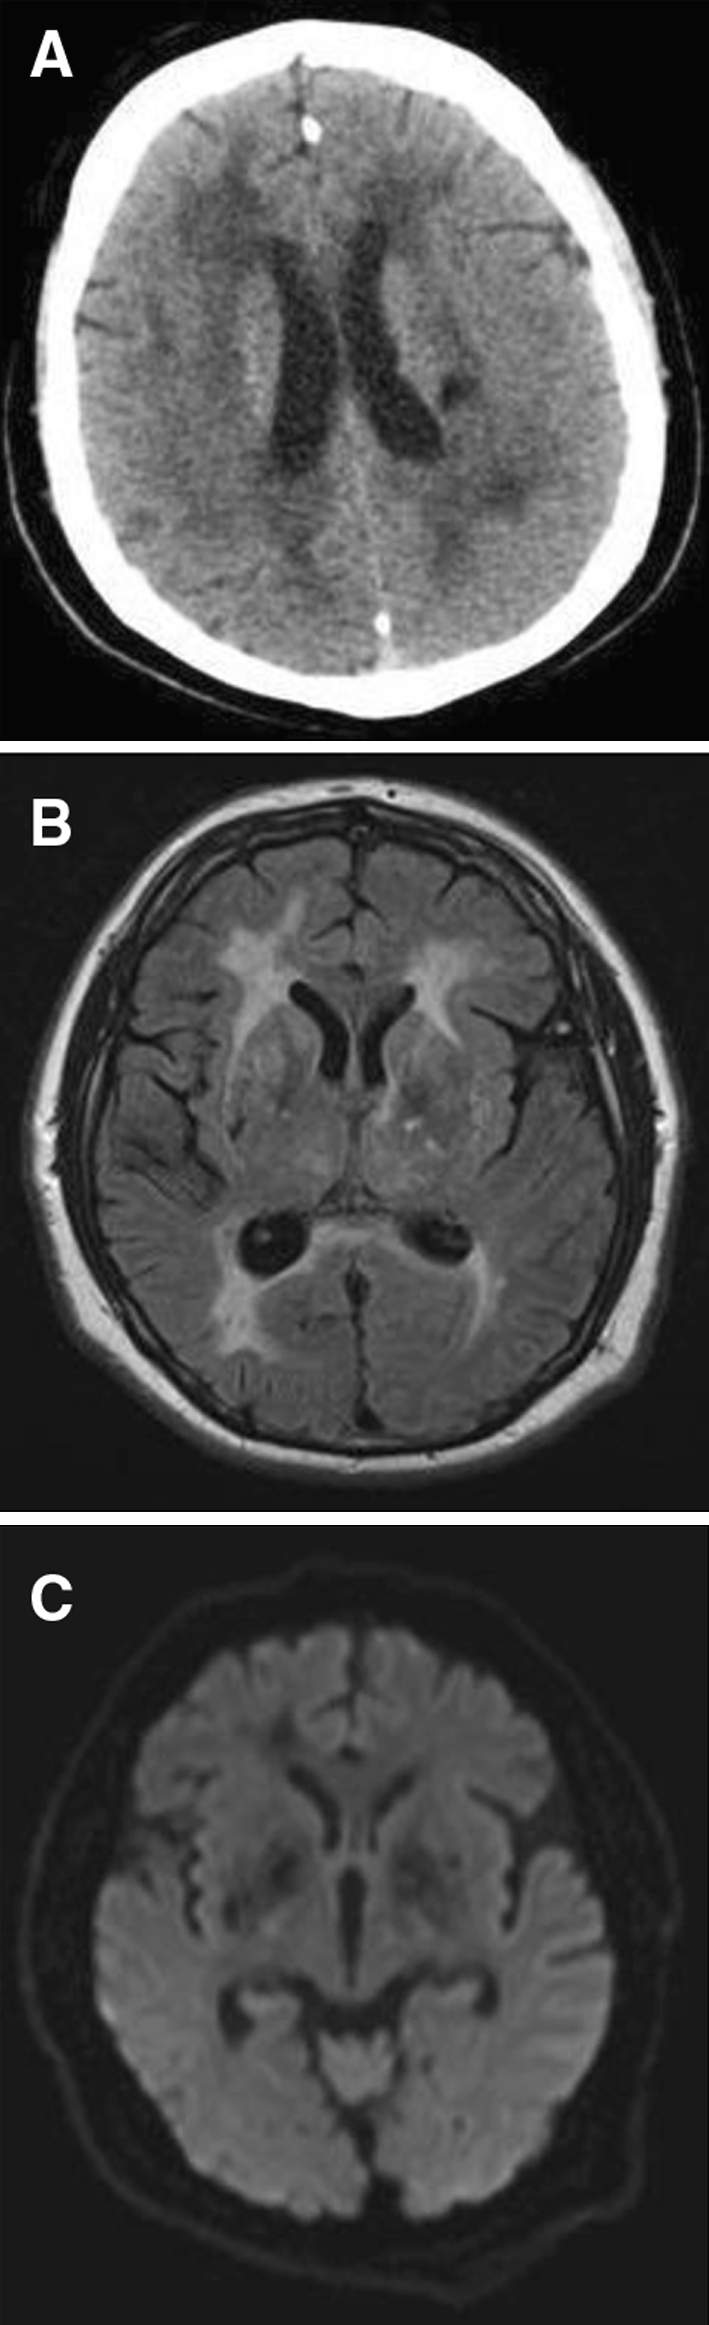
a Confluent symmetric leukoaraiosis typical of CADASIL on admission head CT. b Fluid-attenuated inversion recovery (FLAIR) series in brain MR imaging showed old basal ganglia infarctions in addition to extensive white matter hyperintensities. c Diffusion tensor MR imaging showed no signs of acute ischemia
